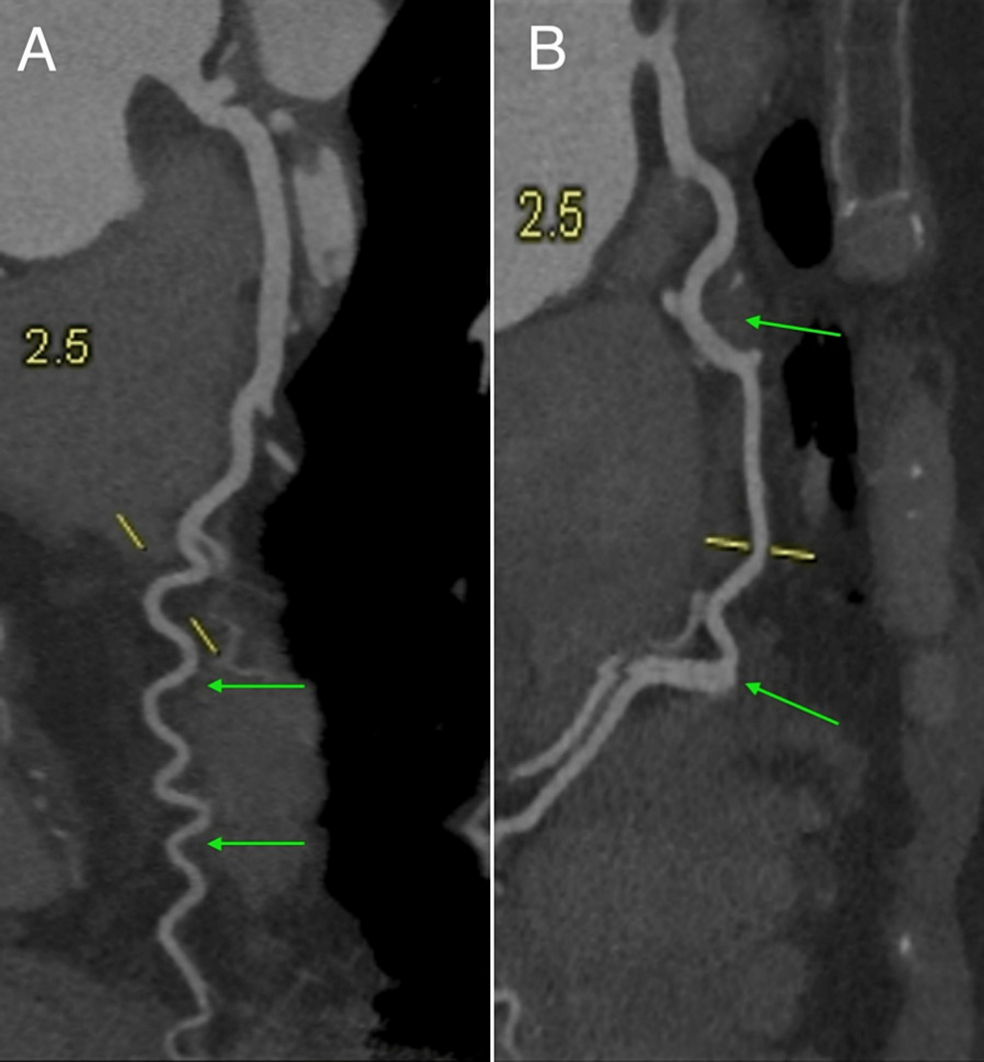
CTA showing (A) significant tortuosity of the left anterior descending and left circumflex arteries (green arrows) and (B) significant tortuosity of the posterior descending artery (green arrows) There were no regional wall motion abnormalities. The ejection fraction is 68%. CTA: computed tomography angiography
